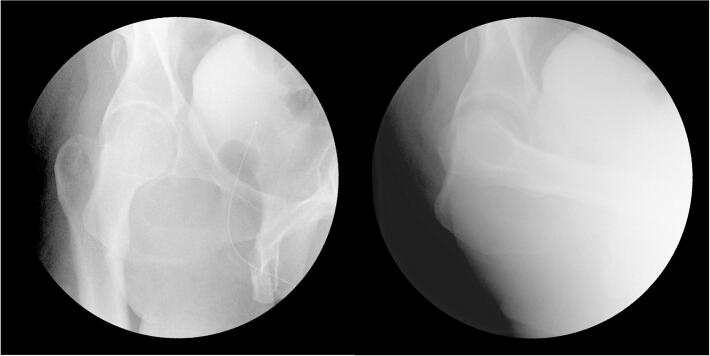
Intraoperative fluoroscopic radiographs taken during the EUA of the right hip demonstrating instability of the right hip on the obturator oblique view with the hip in approximately 60 degrees of flexion.
